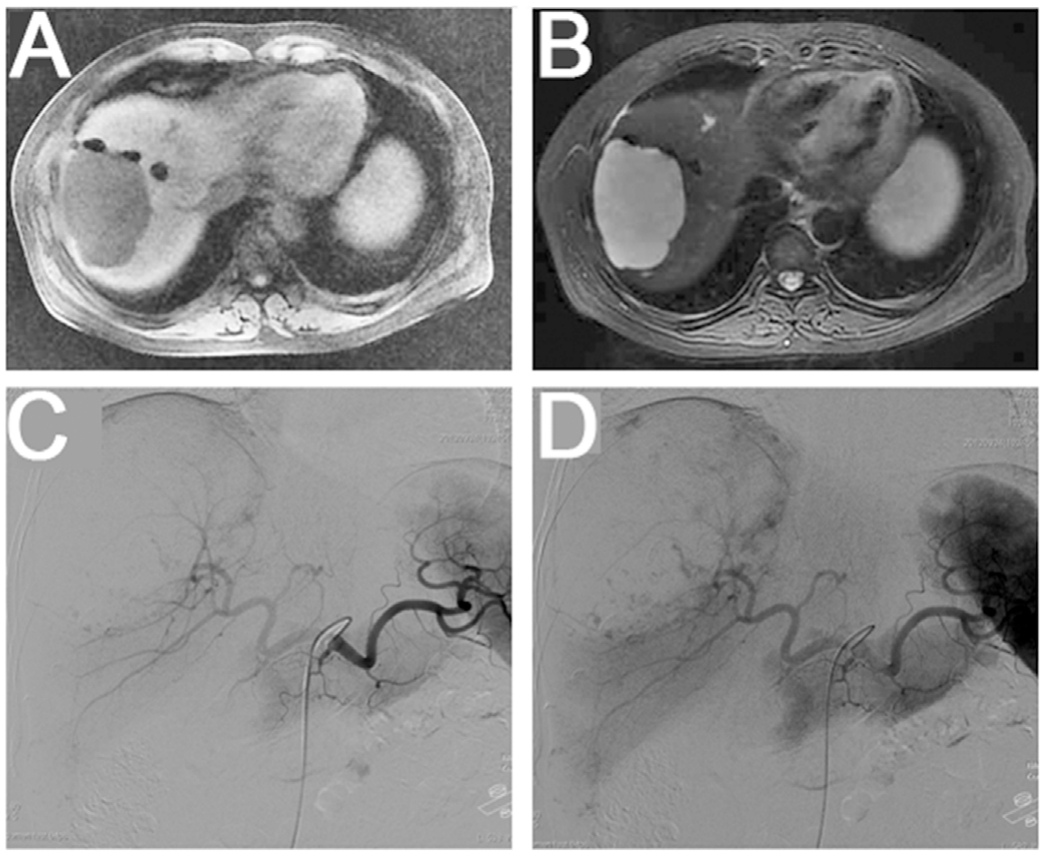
MRI scan. A giant oval mass with low signal in T1WI (A) and high signal in T2WI, appearing as the “light bulb” sign (B). DSA showing intrahepatic massive and multiple nodular tumors, developing in the early arterial phase and gradually filling to the interior, appearing as the “fast out, slow in, tree bearing fruit” sign (C and D).
